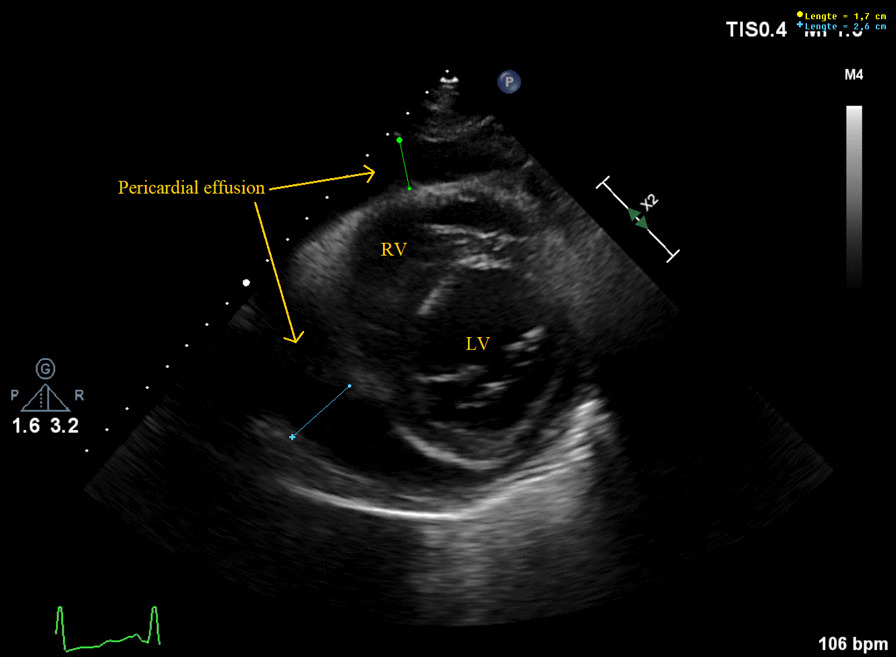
Echocardiogram upon admission (parasternal short-axis view) showing circumferential pericardial effusion. *LV* left ventricle, *RV* right ventricle
